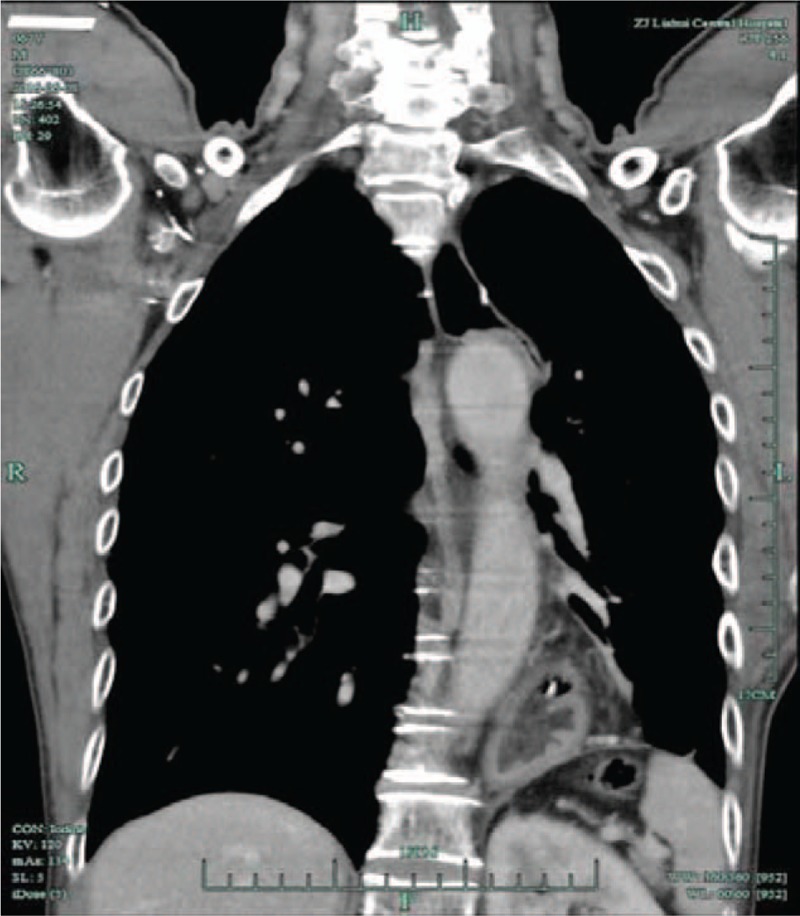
The esophagus and abdominal computed tomography scan is normal before colonoscopy.
